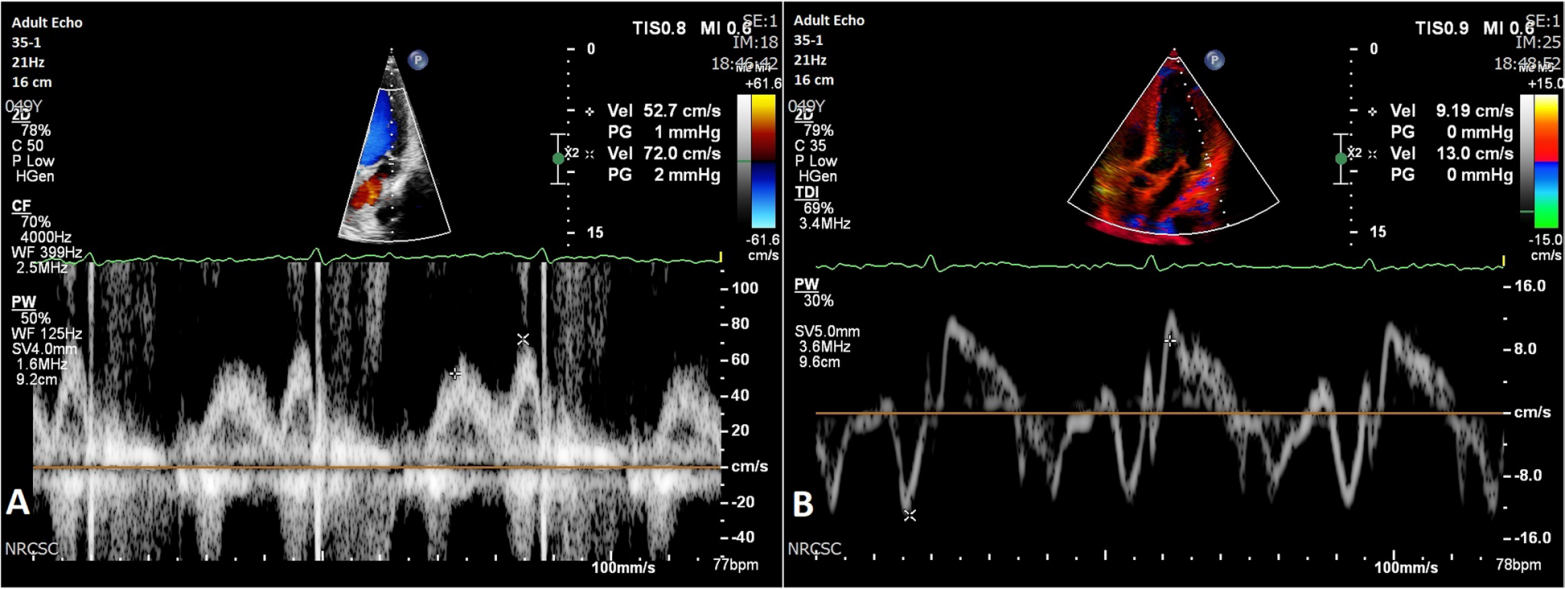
Pulsed wave Doppler echocardiography. Panel A represents the spectral velocity profile at the level of the LV inflow. In Panel B, the sample volume is located at the level of the lateral mitral annulus, which provides velocity information of the myocardium and evaluation of the LV longitudinal function
